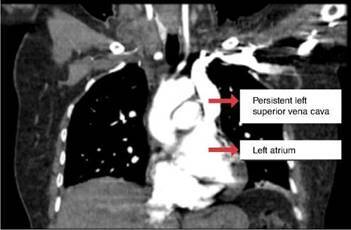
Chest computed tomography angiography showing left superior vena cava draining abnormally into the left atrium
